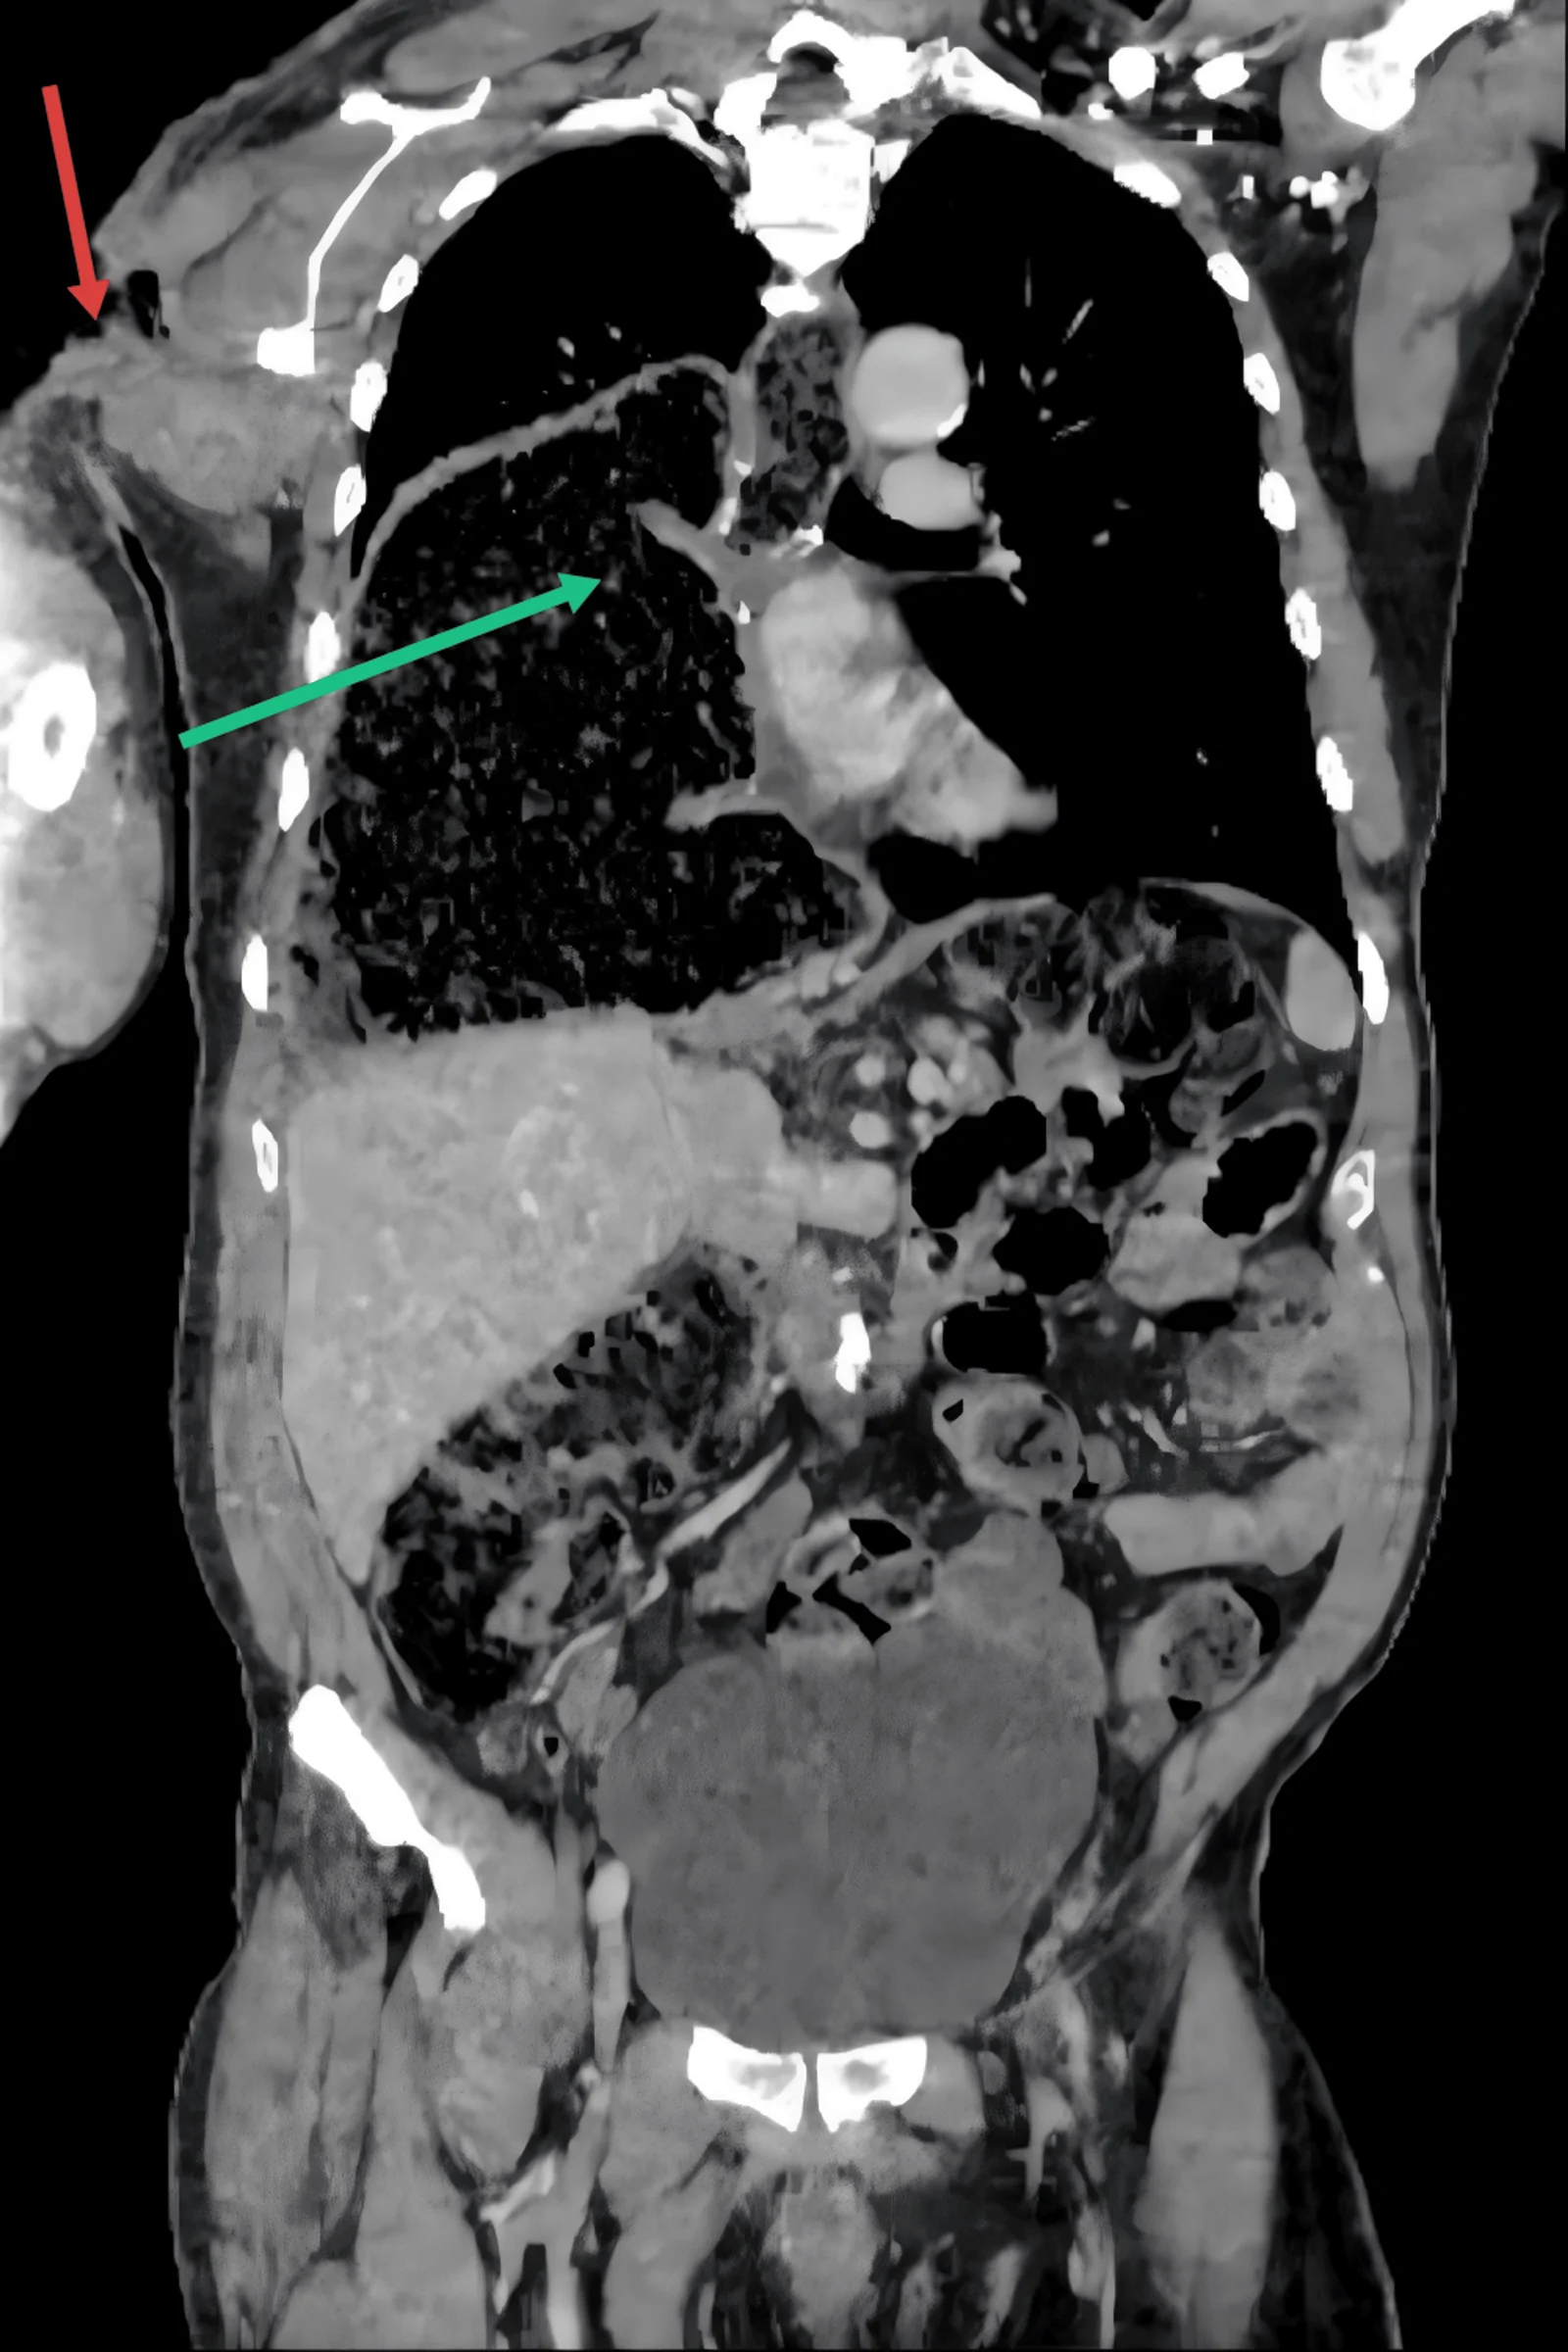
CT of the thorax, abdomen, and pelvis demonstrating postoperative changes and gas (red arrow) within the right posterior shoulder soft tissues. No pulmonary parenchymal abnormality or evidence of disseminated infection is identified. Note postesophagectomy anatomy with gastric pull-up (green arrow) CT: computed tomography
